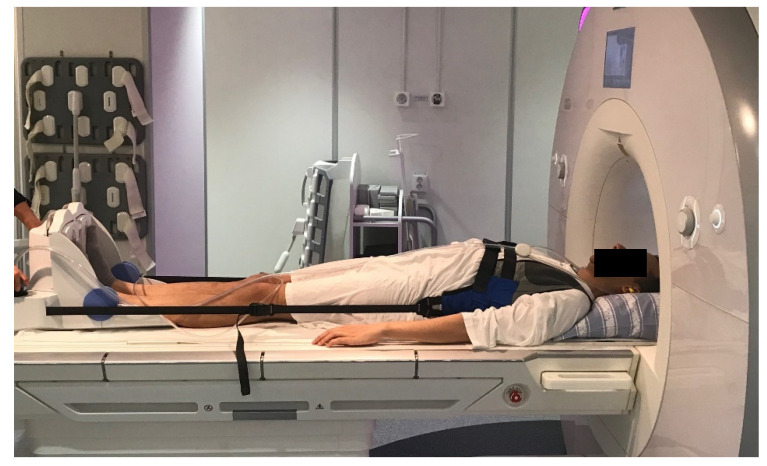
A patient positioned in the Dynawell^®^ compression device just about to undergo magnetic resonance imaging (MRI) examination.
